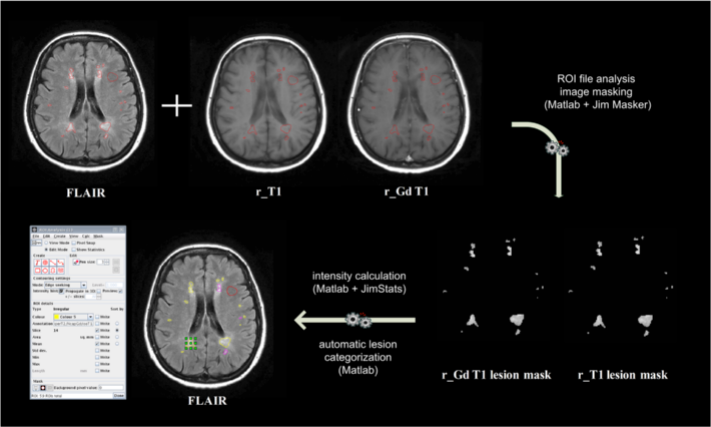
A demonstrative example showing the automatic lesion classification process. FLAIR = axial fluid-attenuated inversion recovery MRI images; r_T1 = registered 2D axial non-enhanced T1-weighted spin-Echo or 3D non-enhanced T1-weighted gradient-Echo MRI images; r_Gd T1 = registered Gadolinium-enhanced T1-weighted spin-Echo MRI images. ROI = Region Of Interest; area used for NAWM mean intensity estimation in red
